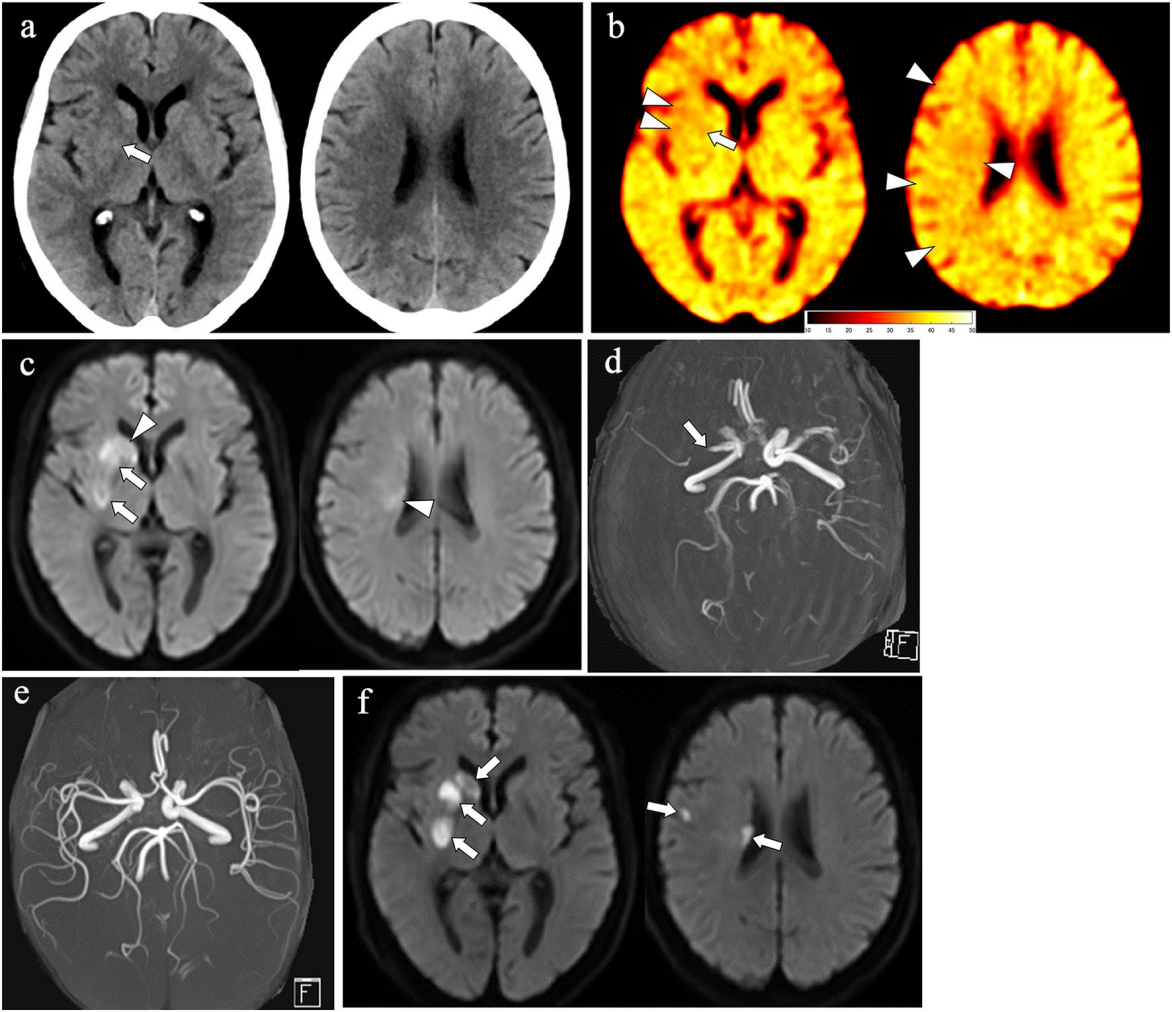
A 77-year-old female with acute right MCA occlusion. Standardized mixed-CT image, X-map, and DWI respectively reveal EIC (a, arrow), hypoattenuation (b, arrow), and hyperintensity (c, arrows) in the right lentiform nucleus (L) indicating acute ischemic infarction, and DWI also reveals hyperintensity in the right caudate nucleus (C) and W segments (c, arrowheads). On the other hand, X-map shows hypoattenuation in the right W segment (b, arrowhead) that is not detected on the mixed-CT image (a), and X-map reveals hypoattenuation in the right I, M4, M5, and M6 segments (b, arrowheads) that is not apparent on the mixed-CT image (a) and the DWI (c). Considering the initial MRA finding of right proximal MCA occlusion (d, arrow), these mismatched lesions on the X-map (b, arrowheads) are included in the right occluded MCA territory without restricted diffusion, i.e., MRA-DWI mismatch area. MRA after reperfusion therapy on day 1 reveals complete recanalization of the right MCA (e). Standardized follow-up DWI (f) on day 1 shows hyperintensity in the right L, C, W, and a small part of M5 segments (arrows), which indicates irreversible acute infarction. The right I, M4, M5, and M6 segments where only the X-map revealed hypoattenuation (b, arrowheads) are almost intact on the follow-up images (f). Thus, the X-map hypoattenuation in the MRA-DWI mismatch area may reflect early minimal change in water content caused by the decreased perfusion pressure, where successful reperfusion can rescue
